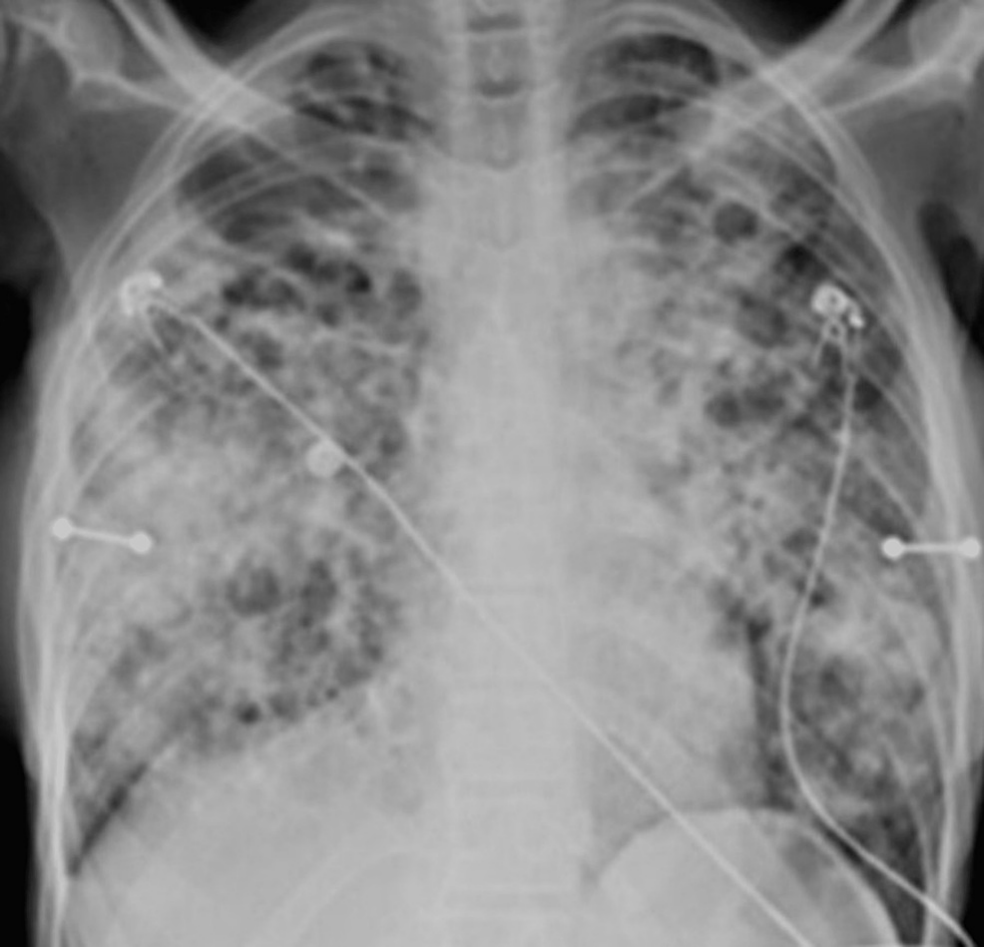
Initial chest X-ray A chest X-ray was taken in the emergency department with diffuse interstitial and airspace opacities bilaterally with interspersed lucencies.
